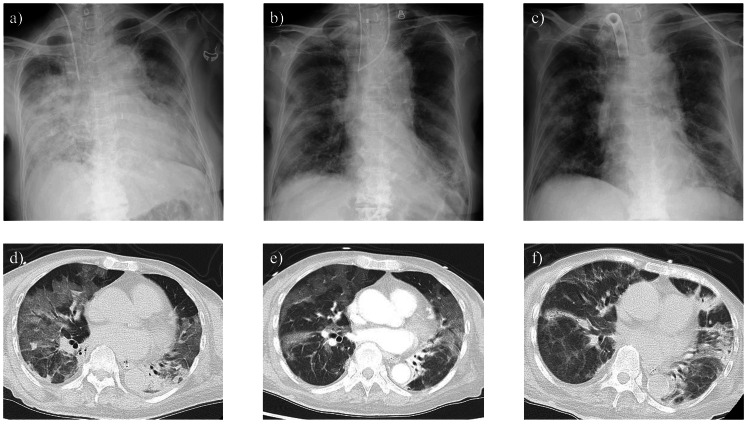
Temporal changes in chest X-rays and chest CT findings. (a,d) Chest X-ray and noncontrast chest CT on day 1, respectively. (b,e) Chest X-ray and contrast-enhanced chest CT on day 11, respectively. (c,f) Chest X-ray and noncontrast chest CT on day 45, respectively CT: computed tomography
